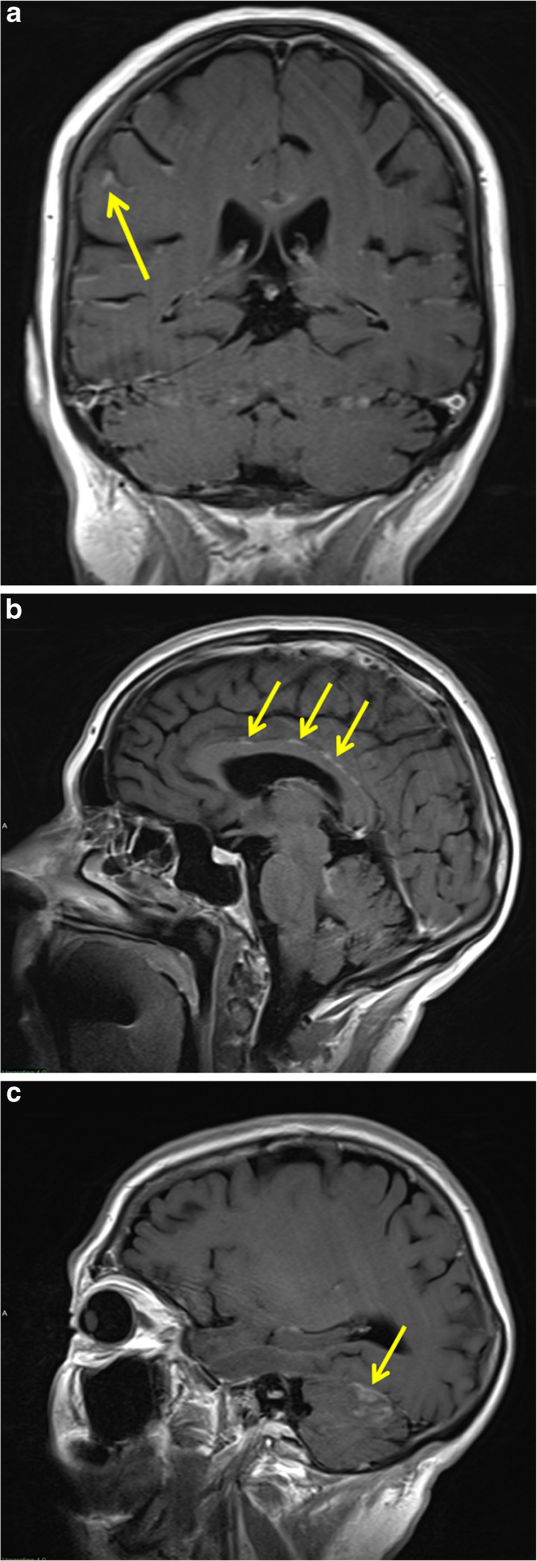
Carcinomatous meningitis in a 67-year-old woman with stage IV non-small cell lung cancer. Coronal (a) and sagittal (b, c) T1-weighted MR images after intravenous gadolinium contrast administration demonstrate diffuse, nodular leptomeningeal enhancement involving both cerebral hemispheres, but more markedly along the tentorium and into the subarachnoid spaces between the cerebellar folia (*yellow arrows*)
